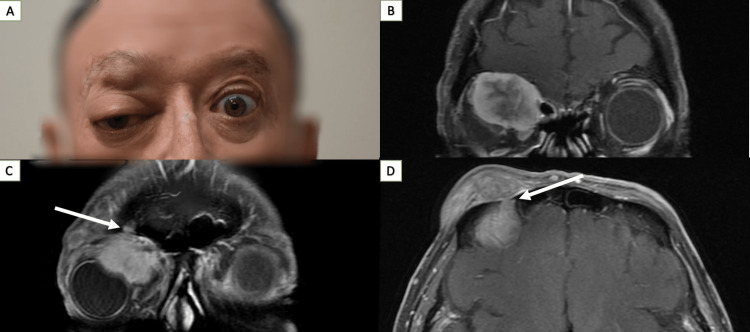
A: Patient with proptosis OD. B-D: MRI of pre-septal mass and intraorbital mass
